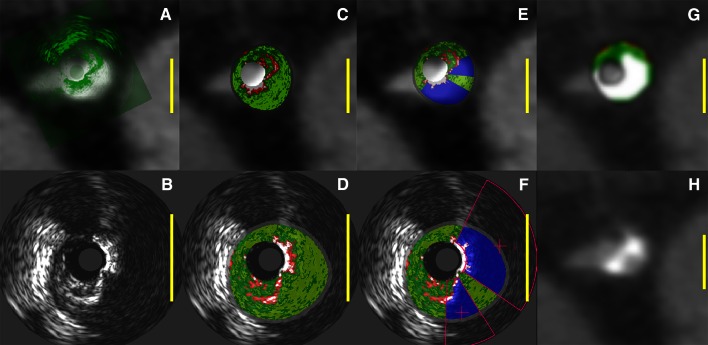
Example of the registered images after fusion. The *yellow scale bars* represent 5 mm. a CTA image with IVUS overlay b in *green* correctly translated and rotated on the CTA image. c Contains the corresponding VH overlay from d and similarly e contains the eVH image from f. The QCT tissue overlay is shown in g from the CTA image in h. Note that all overlays are mirrored in the *top row* for a correct fusion. The overlays in C–G have five color codes: *red* (NC), *light*-*green* (FF), *dark*-*green* (FI), *white* (DC), and *blue* for the *masked areas*. There is a nice correspondence between the masked areas in e and the two calcified areas with high intensities in h with in between a calcified area with lower intensity, more similar to the luminal contrast intensity in the bifurcating artery. This example shows that the DC area in VH is underestimated when compared to the DC area in the tissue overlay in g. Adding the blue quantified acoustic shadow to the total DC volume in eVH will approximate the DC volume in QCT
